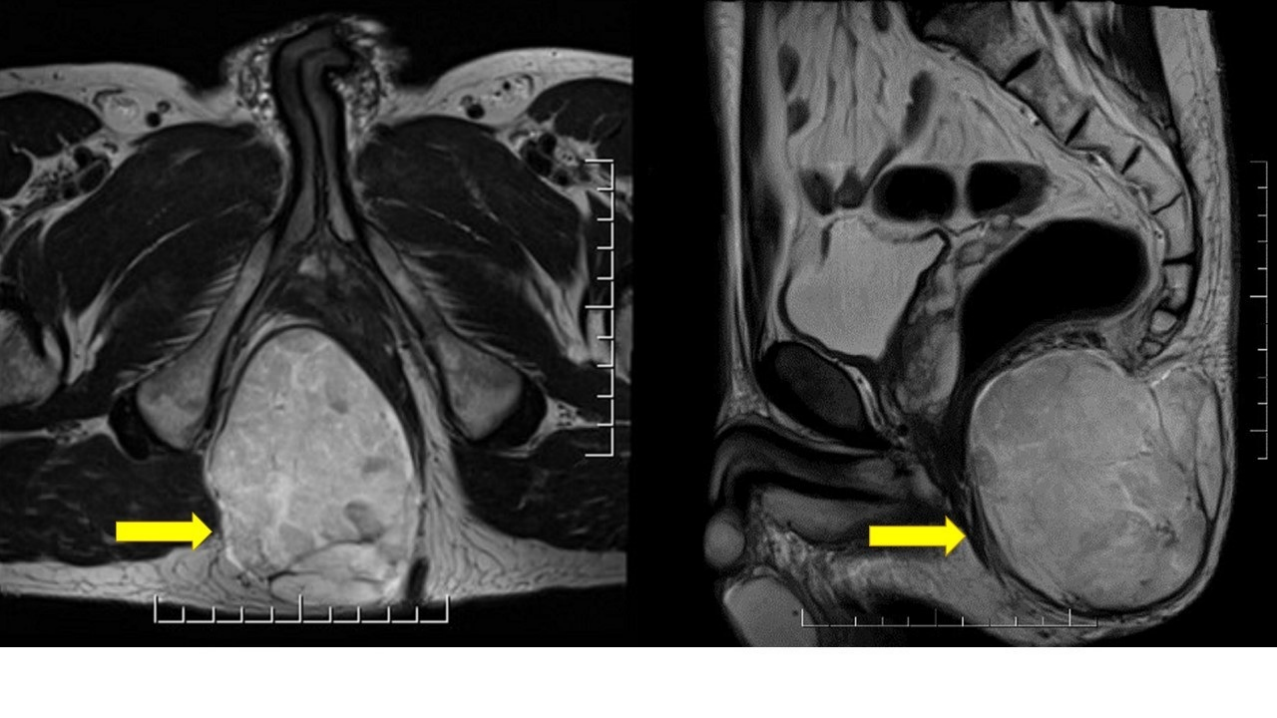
Axial and sagittal T2-weighted magnetic resonance imaging (MRI) sequences showing a multilobulated mass with heterogeneous enhancement
